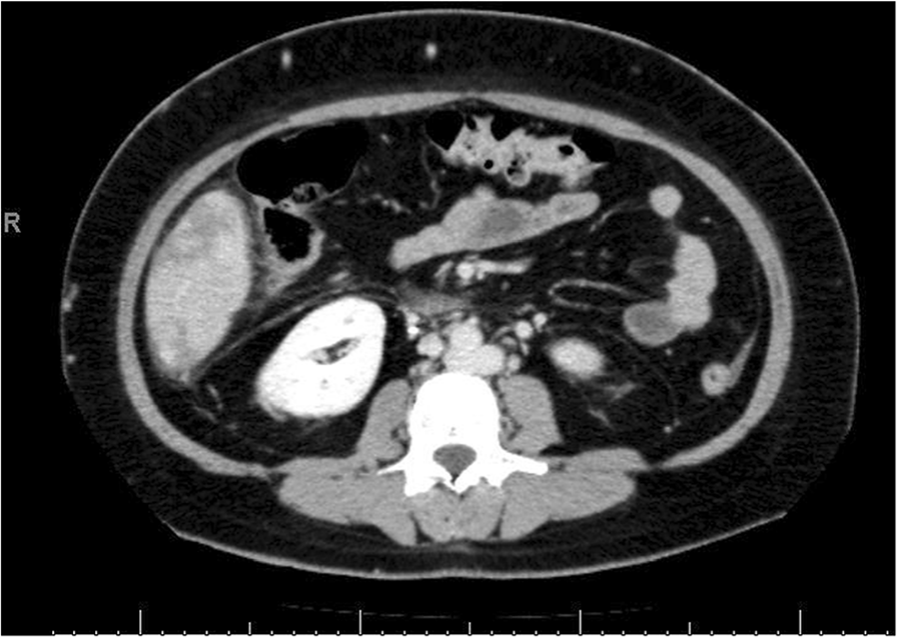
Computed tomography scan showing an abnormal perfusion with multiple small ill-defined hypodensities of the right hepatic lobe.
